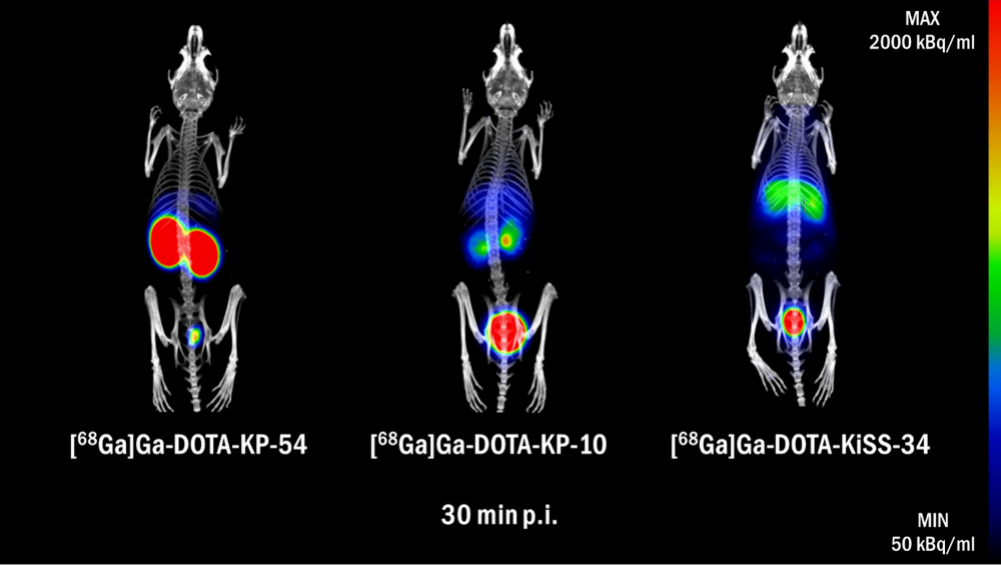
PET/CT images of healthy
